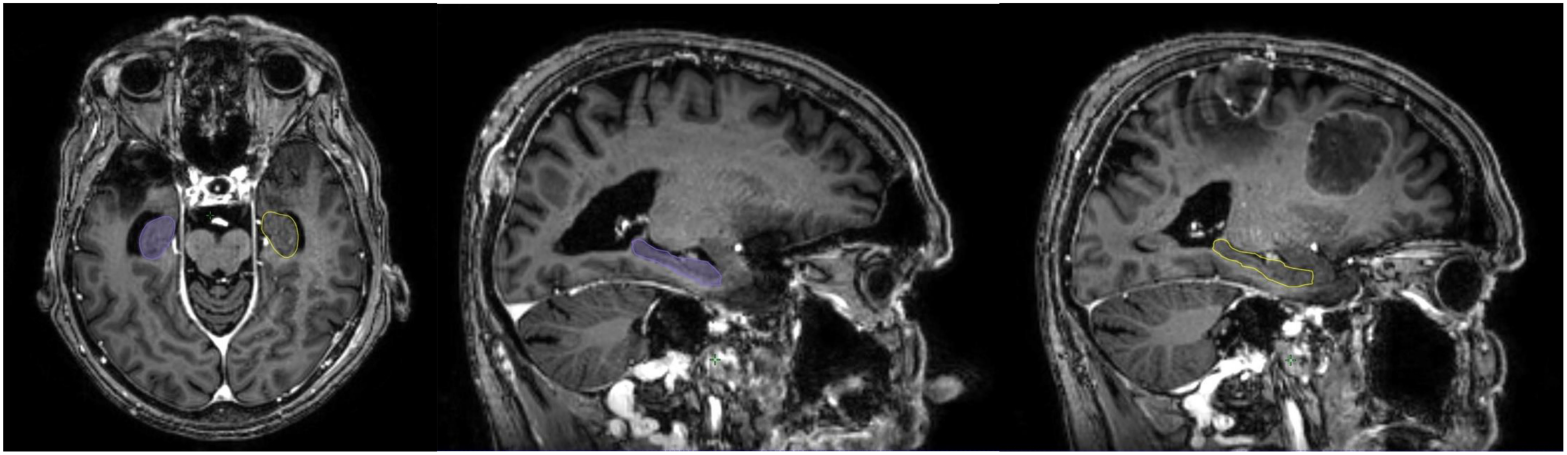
MR image with hippocampus contouring.
